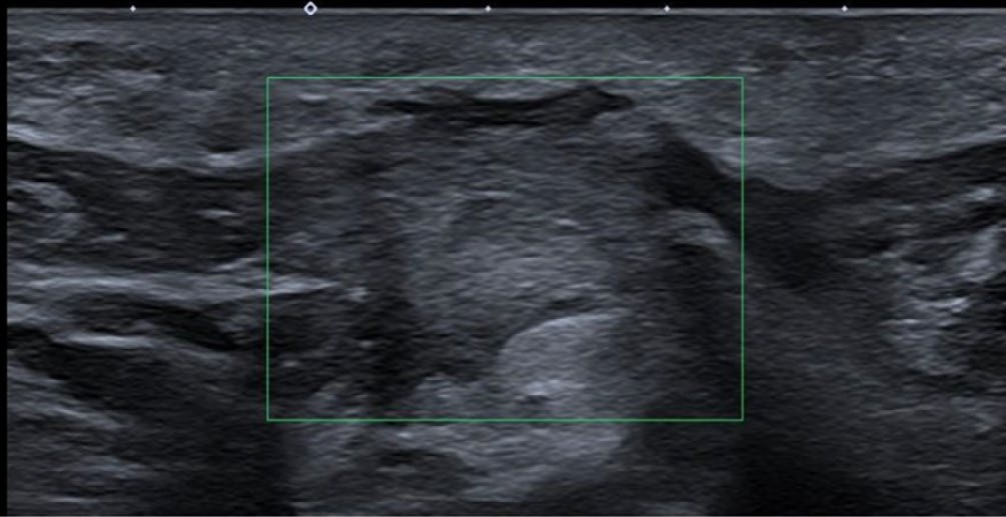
Postoperative hematoma on HFUS. The image shows an ill‐defined, heterogeneously hypoechoic area in the subcutaneous tissue, compatible with early organizational blood collection. No internal vascular signal is detected on colour Doppler, confirming non‐perfused content. Over time, these lesions typically decrease in size and echogenicity, differentiating them from abscesses or seromas by their inhomogeneous echoes and irregular contours.
